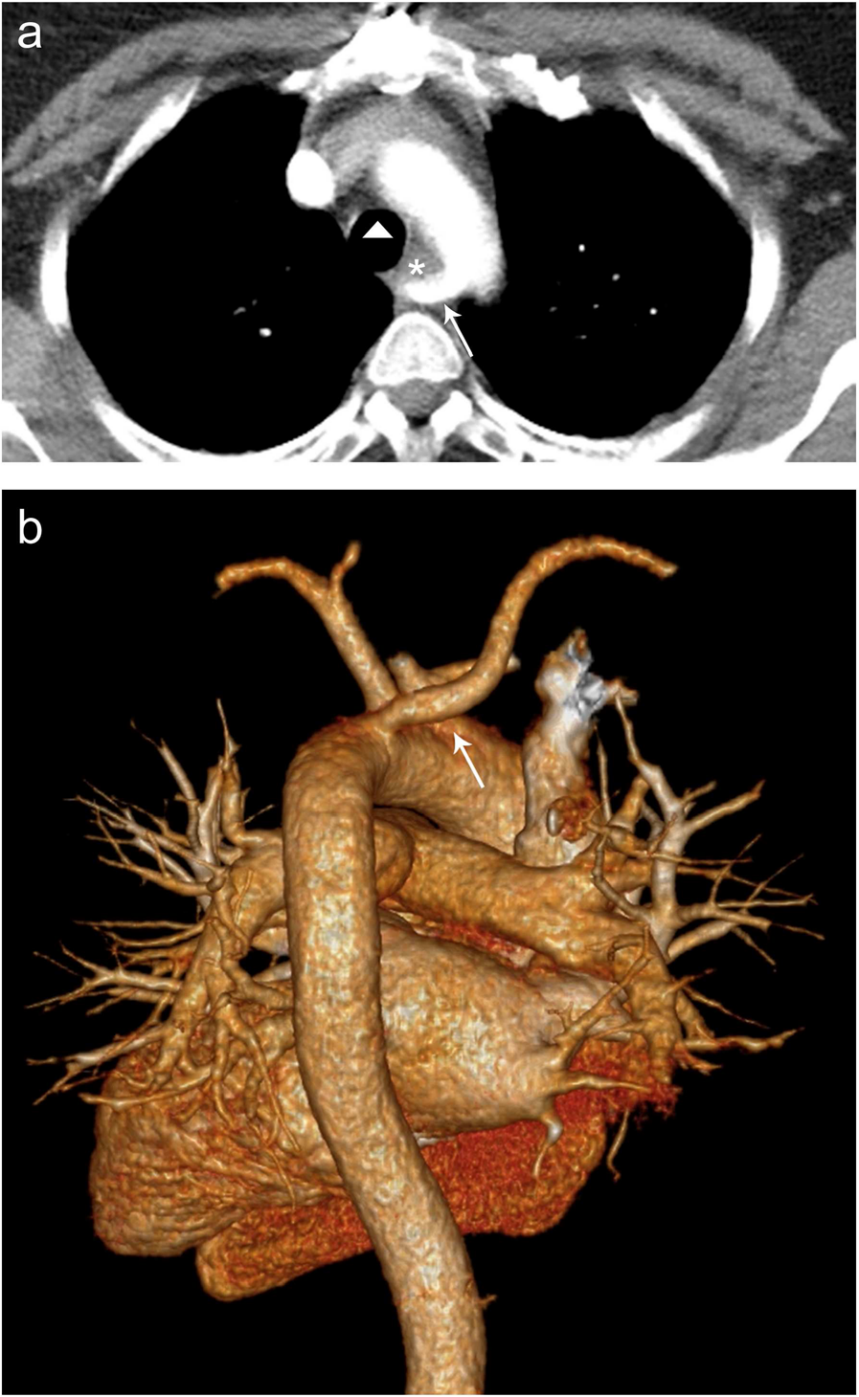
Aberrant right subclavian artery. Axial (a) CT image demonstrating aberrant right subclavian artery (arrow) coursing posterior to the esophagus (asterisk) and trachea (triangle). b 3D model demonstrating the distal take-off the aberrant right subclavian artery (arrow) from the aortic arch
